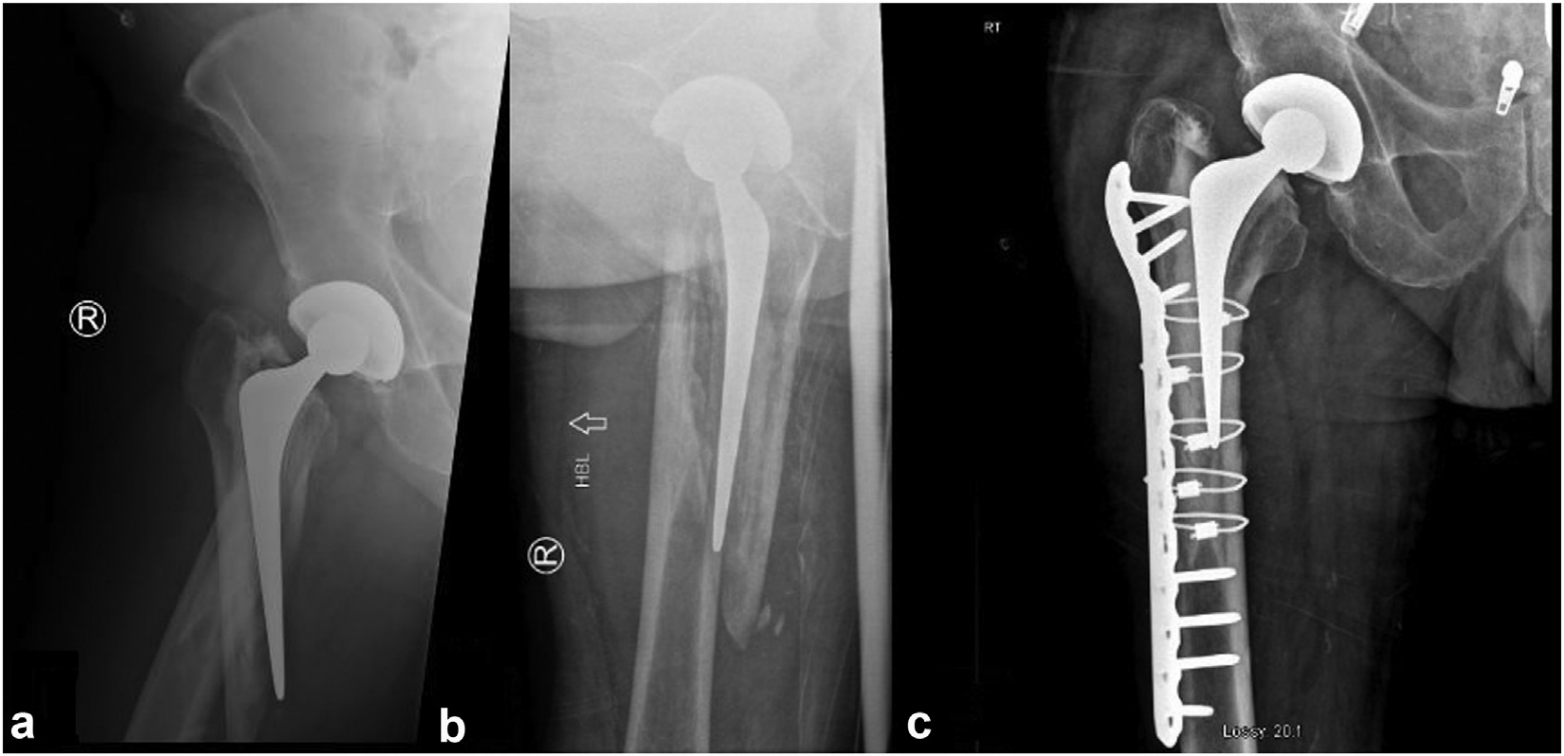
(a) Anteroposterior radiograph of the periprosthetic fracture in a THA. (b) Vertical orientation of the cross table lateral radiograph. (c) The same patient after cement-in-cement with ORIF revision to a short Exeter stem.
